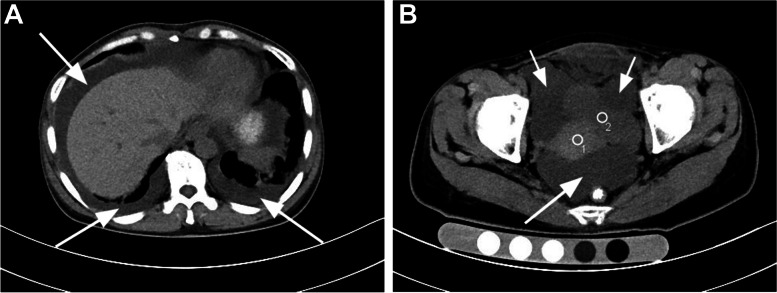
A and B, CT coronal images through the 10th thoracic vertebrae and the pelvis demonstrate there were multiple irregular cystic and solid masses in bilateral adnexa (White small arrow). A large amount of effusion (white big arrowhead) is present within the bilateral thoracic cavity and pelvic and abdominal cavity. the uterus (circle 1), the bladder (circle 2)
